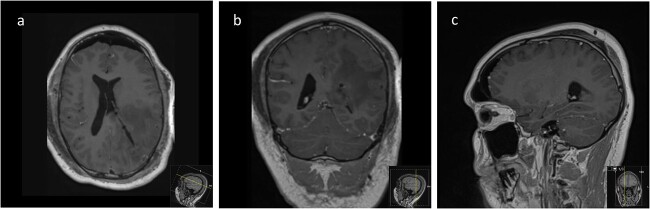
Post-operative axial (a), coronal (b), and sagittal (c) T1-weighted post-gadolinium MRI sequences of the Patient showing complete resection of the lesion with no evidence of post-operative surgical complications and complete collapse of the operative corridor pathway after removal of the tubular retractor.
